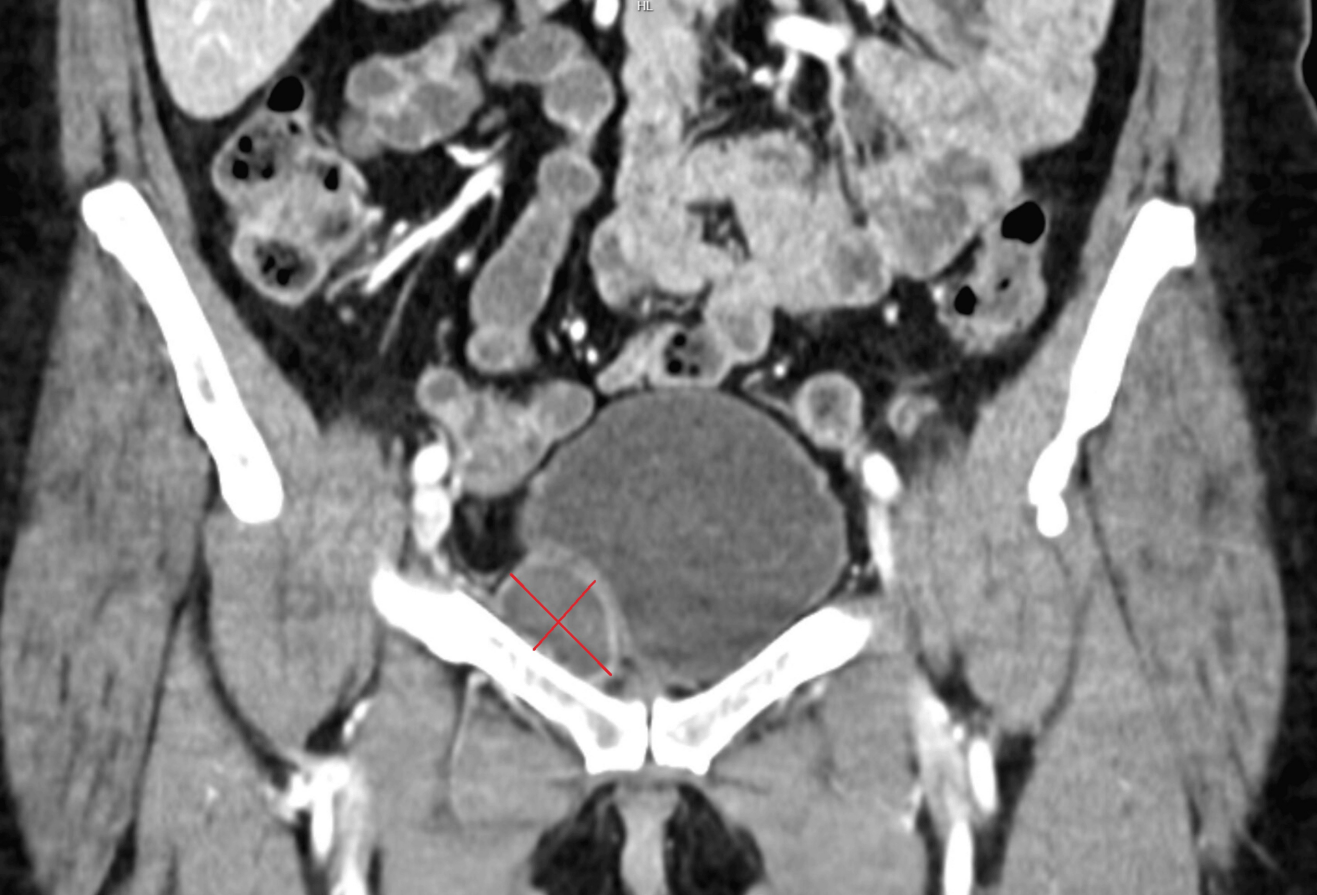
A follow-up CT scan two months after the initial treatment revealed a smaller, organized, hypodense hematoma. At that time, the patient did not report any pain.
